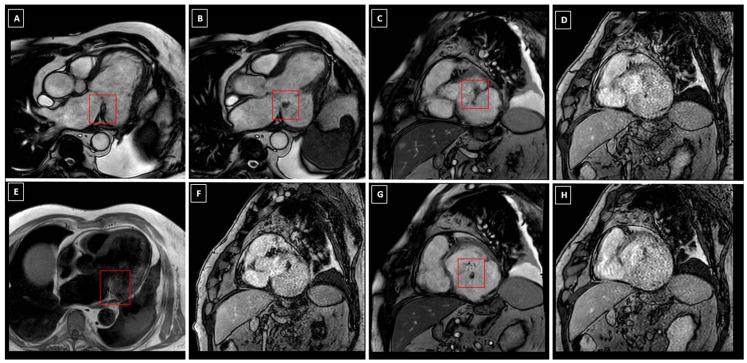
Cardiac magnetic resonance (CMR) features of complicated CCMA. (A,B) show bright-blood balanced steady-state free precession (b-SSFP) sequence in two different slices in three-chamber view. (C,G) display b-SSFP sequence in short axis view, at the mitral annulus level (C) and at the left ventricular basal level (G); corresponding slices acquired by inversion recovery gradient-echo (IR-GRE) late gadolinium enhancement (LGE) sequence with long inversion time (600 ms) to null thrombus signal are showed in (D,H), respectively. (E) shows black-blood T1-weighted fast spin echo (FSE) sequence, while (F) displays IR-GRE LGE sequence acquired with standard inversion time of 250 ms. Red frame indicates ruptured caseous calcification of the mitral annulus with leaking caseous material.
